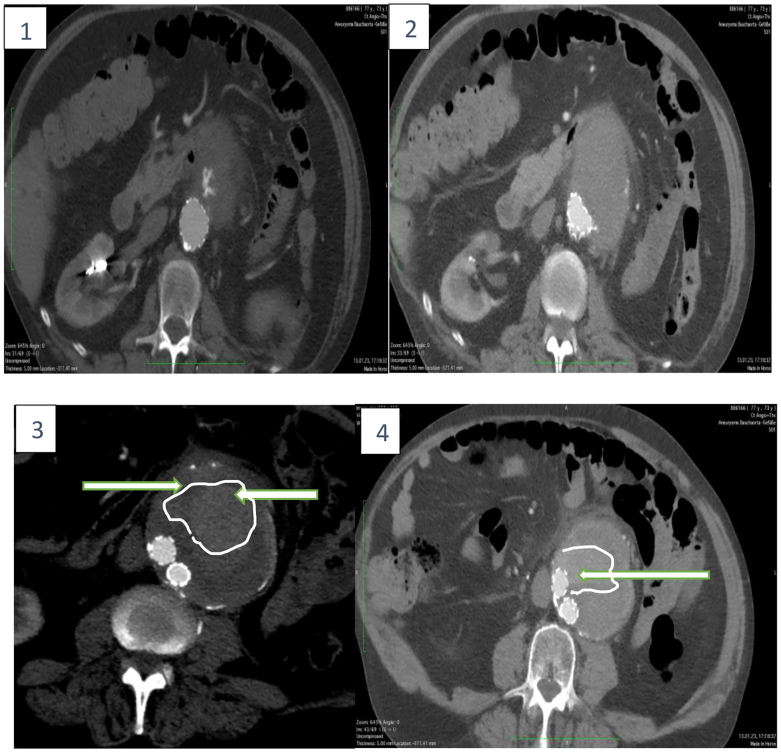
Computed tomography (CT) scan at admission: patient 1.
